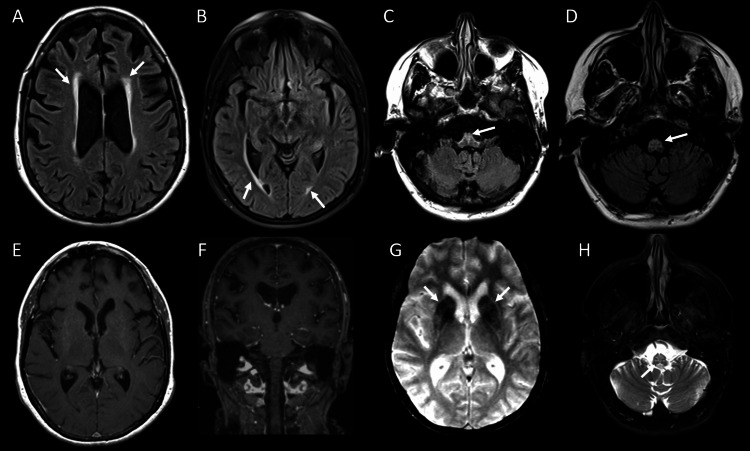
Contrast-enhanced MRI of the brain Brain MRI demonstrates mild to moderate generalized brain volume loss with ex-vacuo prominence of the ventricles and cerebral sulci. On the T2-FLAIR sequence, there is mild diffuse periventricular rim FLAIR hyperintensity (arrows on A and B) without deep white matter involvement. At the level of the medulla and upper cervical spine, there is moderate atrophy and increased T2/FLAIR signal (arrows on C, D, and H) without a mass configuration. Postcontrast imaging (E and F) demonstrates no abnormal enhancement in the basal ganglia, white matter, or brainstem. T2*GRE (G) demonstrates hypointensity, suggesting prominent mineralization in the basal ganglia, particularly in the caudate nucleus and putamen (arrows on G) without atrophy. FLAIR: fluid-attenuated inversion recovery; GRE: gradient recalled echo
